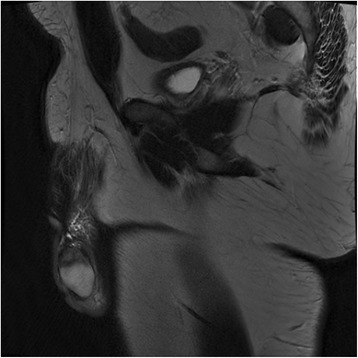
Contrast-enhanced magnetic resonance imaging, T2-weighted images showing a heterogenic hypointensity in the upper part of the testicle
